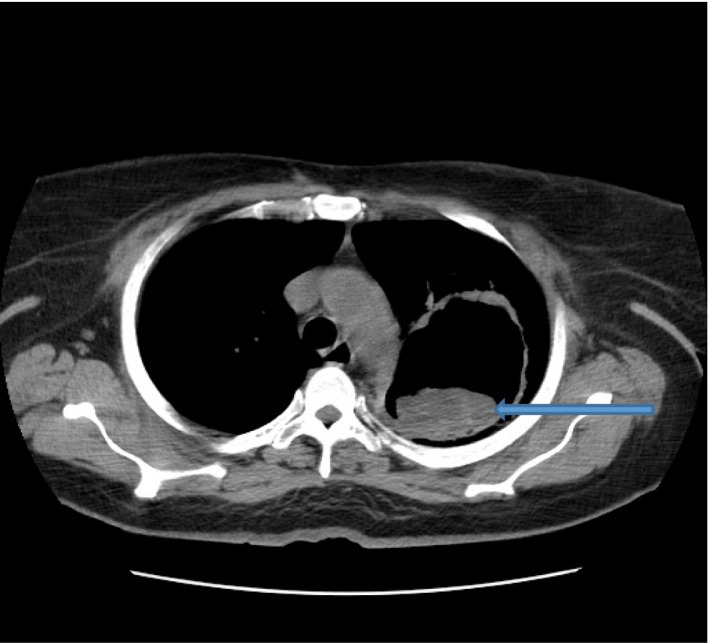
Chest CT scan showing a cavity with a hyperdense shadow in the dependent area.
